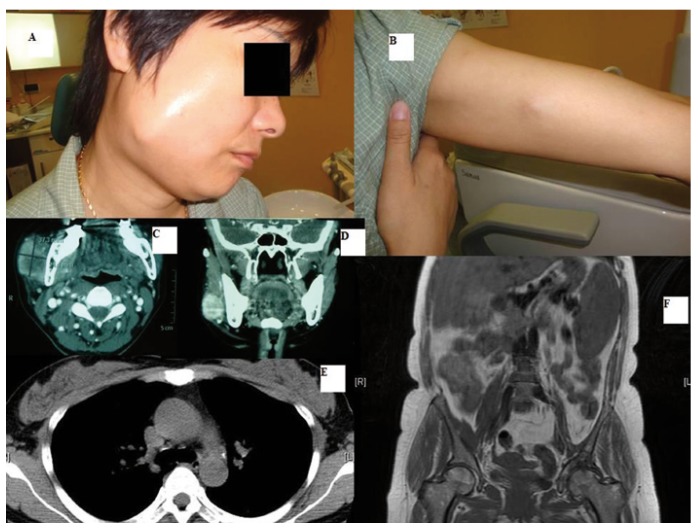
A, B) clinical images of metastases in the right subcutaneous paramandibular region and on the left forearm of a cervical squamous cell carcinoma. C, D) Axial and coronal CT views of the right paramandibular metastasis not involving the skin, mucous or bone. E) Thoracic CT showing small nodules suggestive of pulmonary metastasis. F) Coronal abdominal MR showing squamous cell carcinoma of the cervix, confirmed by biopsy.
